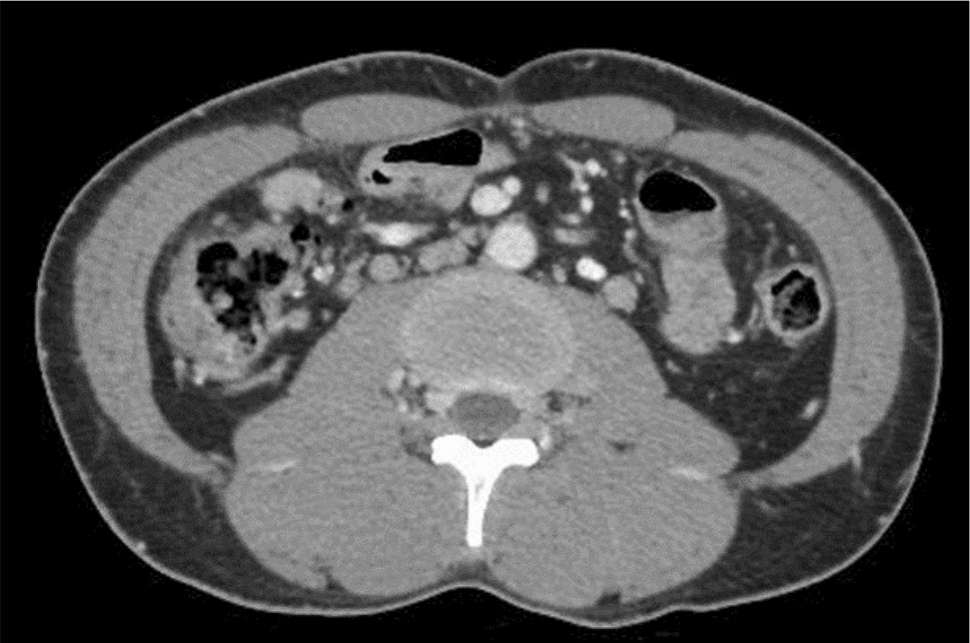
Abdominal contrast computed tomography performed in 2015
